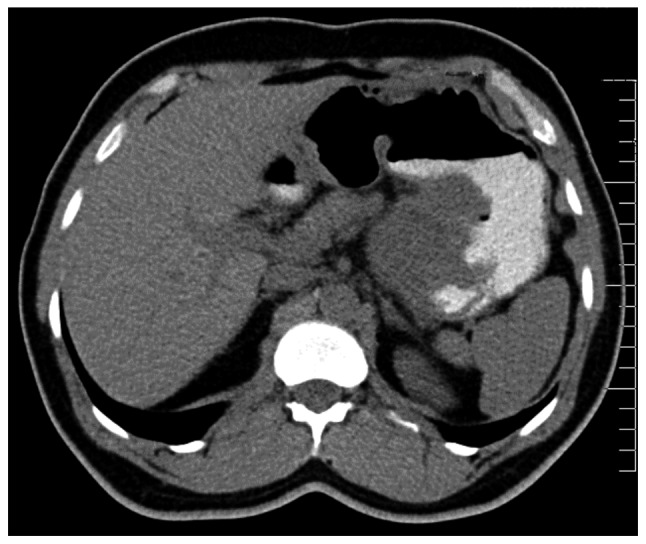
Computed tomography scan revealing an intestinal gastrointestinal stromal tumor measuring 10×15×9 cm.
